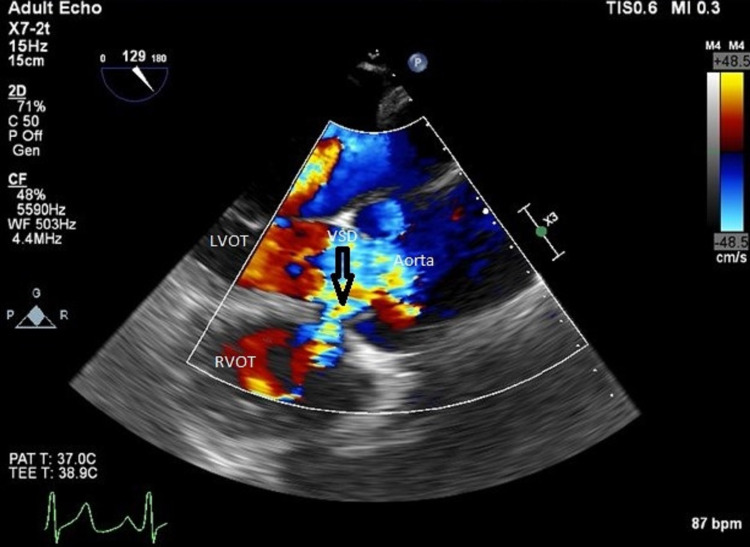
Transesophageal echocardiogram with color flow showing the left to right shunt (from the left ventricle to the right ventricle) through the outlet VSD LVOT: left ventricular outflow tract; RVOT: right ventricular outflow tract; VSD: ventricular septal defect
